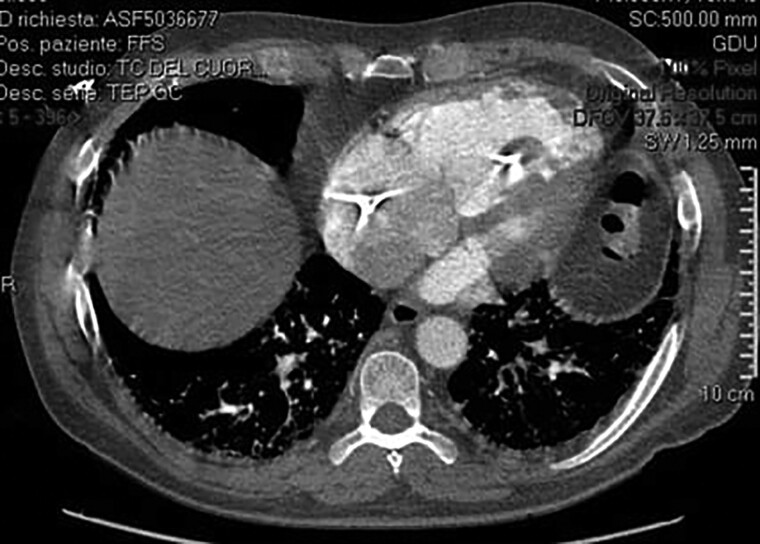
Computed tomography of the heart. A voluminous mass (area 2.6 cm^2^) on right ventricle pacing lead.
